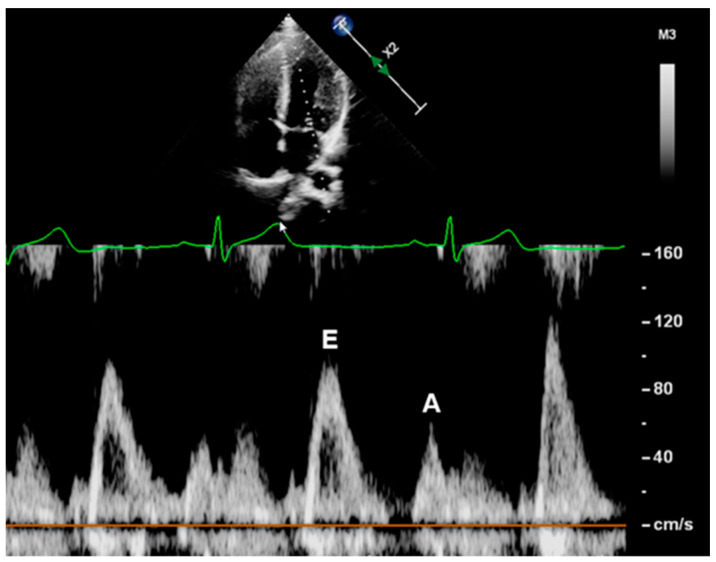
Measurement of mitral inflow velocities. The sample volume of a pulsed-wave Doppler was placed at the tip of the mitral valve in an apical four-chamber view. The mitral peak flow velocities at early (E, cm/s) and late (A, cm/s) diastole were measured offline.
